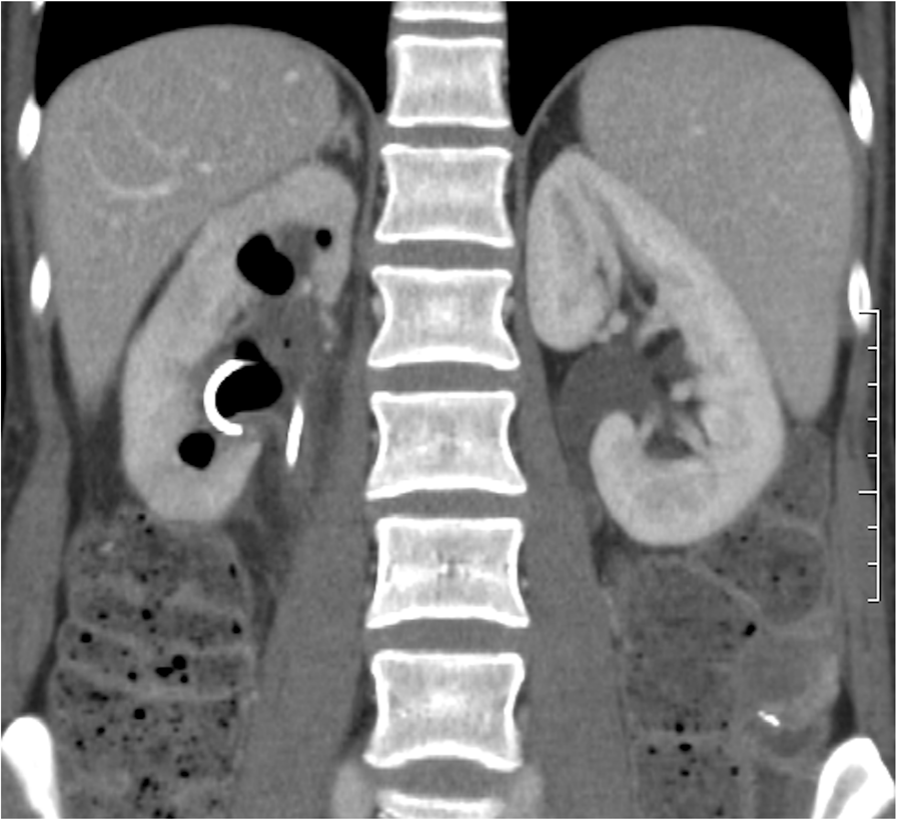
Coronal section of the CT scan in a late-venous phase revealing presence of gas inside the right pyelo-calyceal system and the upper coil of the double J stent
